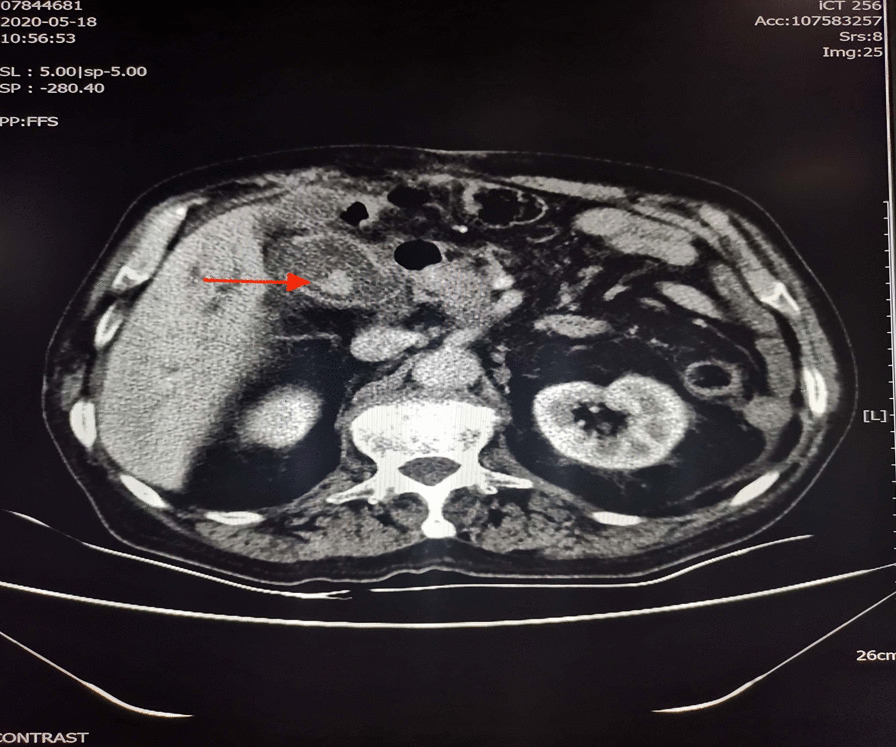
Abdominal CT: enlarged gallbladder, thickened wall and circular high-density shadow
